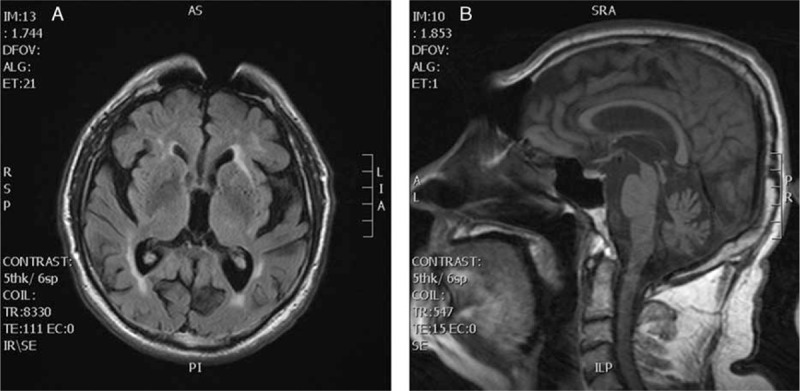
Horizontal FLAIR MRI sequence showing diffuse brain atrophy with postischemic hyperintensities in white matter bilaterally and nonhomogeneous signal and blurred contours of the basal ganglia (A). Sagittal T1-weighted MRI showing regular configuration of the brainstem without noticeable atrophy (B). FLAIR = fluid-attenuated inversion recovery, MRI = magnetic resonance imaging.
